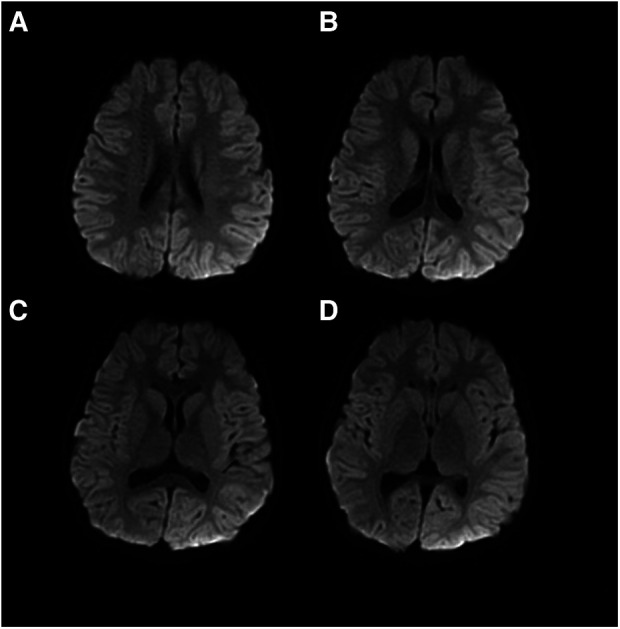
The brain MRI of Patient #1 showed complete disappearance of abnormal signals 1.5 months after treatment (A–D).
